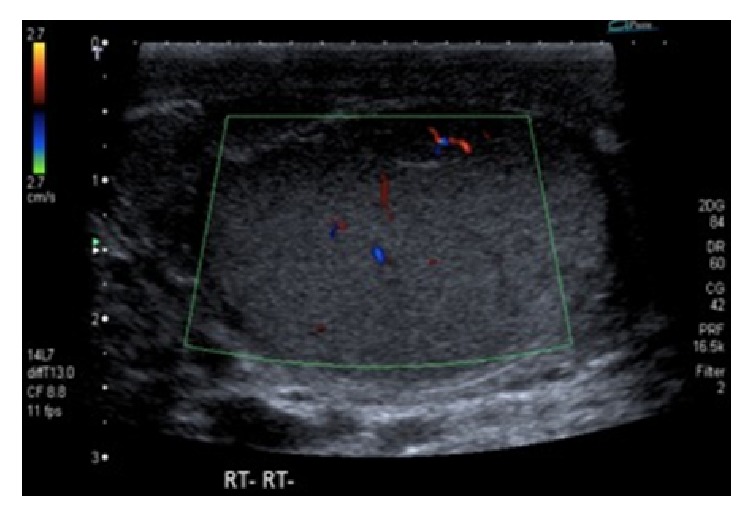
Follow-up ultrasound scan of right testis with normal perfusion.
